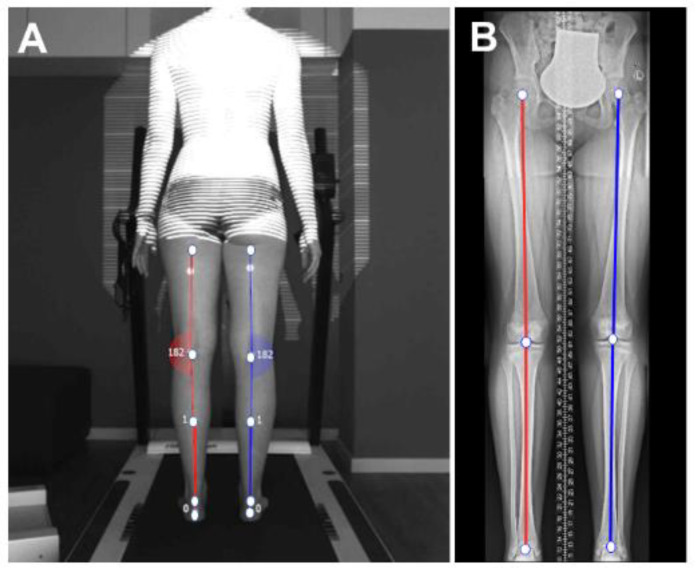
Representation of (A) Motion capture analyses (MCA): non-invasive 2D motion capture leg axis evaluation (Diers leg axis posterior^®^). Markers are placed on five anatomical landmarks of the skin (gluteal rim, middle of the popliteal fossa, middle underneath the bellies of the musculus gastrocnemius, calcaneus top edge and calcaneus lower edge) and leg axis analysis is performed; and (B) long-standing full-leg radiograph (LSX): mechanical axis angle (MAA_rad_) is defined by the line from the hip center to the knee center and the line from the knee center to the ankle center.
